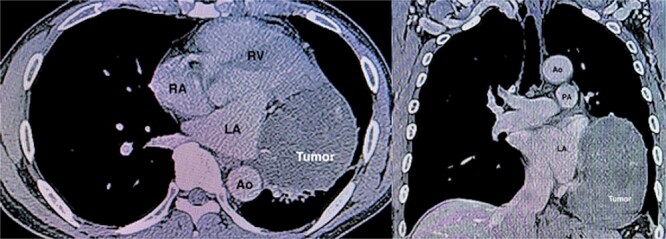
CT angiography demonstrating a huge tumor (8.9 × 7.3 × 12.2 cm) measuring adjacent to the left atrium (LA) and left ventricle (LV). Ao, ascending aorta; RA, right atrium; RV, right ventricle; PA, pulmonary artery.
